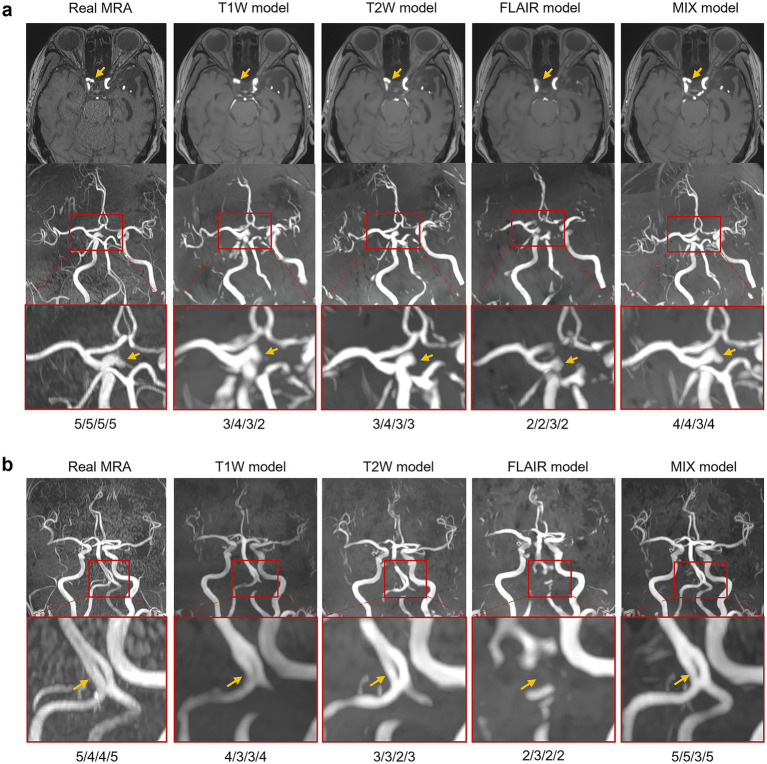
Comparison of synthetic MRA images generated by different Models. (a) Female, 76 years old, real MRA demonstrated an aneurysm at the right internal carotid artery C5 segment. T1W, T2W, and MIX model syn-MRA accurately identified the aneurysm, but T2W model showed a smaller size, and FLAIR model failed to display the aneurysm (yellow arrow). (b) Male, 81 years old, basilar artery fenestration was observed in the real MRA. While T1W, T2W, and MIX model syn-MRA successfully reconstructed the fenestration, FLAIR model failed (yellow arrow). The red box indicates the magnified image, n/n/n/n means overall image quality/vascular edge sharpness/venous contamination/diagnostic confidence scores. MRA = magnetic resonance angiography, T1W = T1-weighted; T2W = T2-weighted, FLAIR = fluid attenuated inversion recovery.
